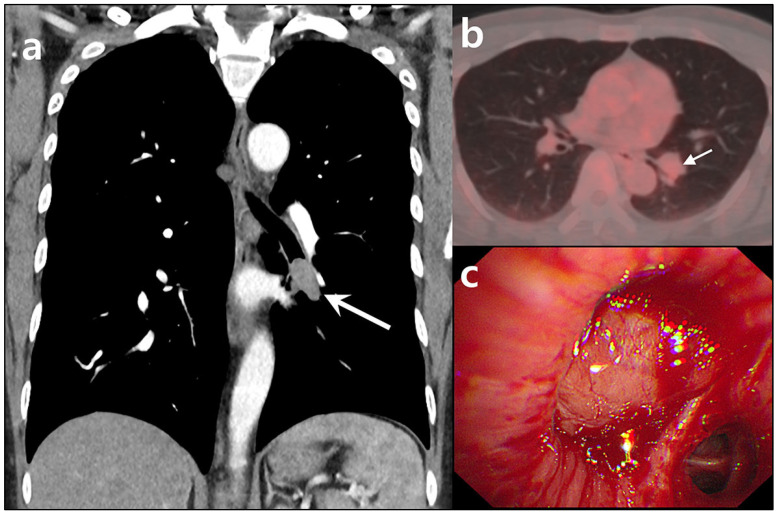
CT, PET/CT, and bronchoscopic images of a typical carcinoid tumor in the left lower lobe in a 40-year-old man. The patient reported experiencing intermittent blood-tinged sputum, which had started three months before presentation. He had a 20 pack-year smoking history and was on medication for hypertension. (a) Chest CT revealed a well-enhancing nodule measuring approximately 2.5 cm, located in the left lower lobe bronchus (arrow). (b) FDG-PET/CT showed the lesion to be mildly hypermetabolic, with an SUVmax of 2.6 (arrow). (c) Bronchoscopy identified a hypervascular, polypoid lesion obstructing the left lower lobe bronchus. A bronchoscopic biopsy indicated a carcinoid tumor. The patient subsequently underwent video-assisted thoracoscopic surgery (VATS) for a left lower lobectomy.
